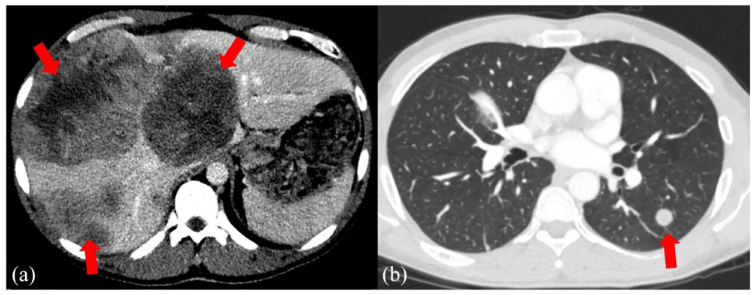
(a) Computed tomography (CT) scan of the abdomen and pelvis: Liver enlargement and multiple heterogeneous hypodense masses depicted with arrows (largest 11 cm). (b) CT scan of the chest: Multifocal left lower lobe pulmonary nodules depicted with arrow (largest 1.6 × 1.4 cm) and pulmonary emboli.
